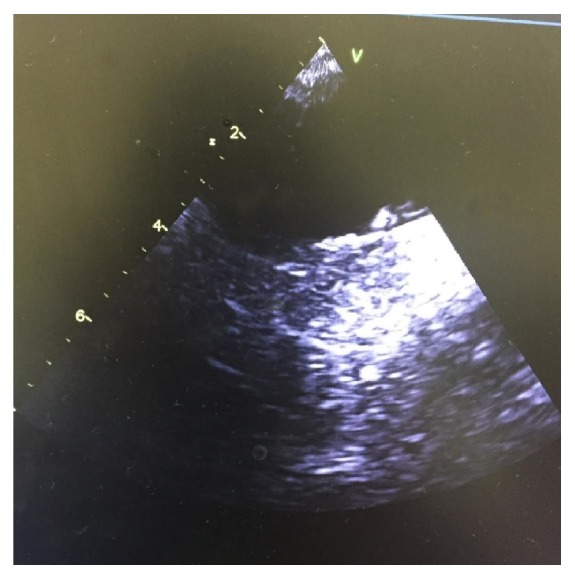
Follow-up TEE performed four months later demonstrating that prior echogenic mass in the aortic arch had decreased in size.* Abbreviation.* TEE: transesophageal echocardiogram.
